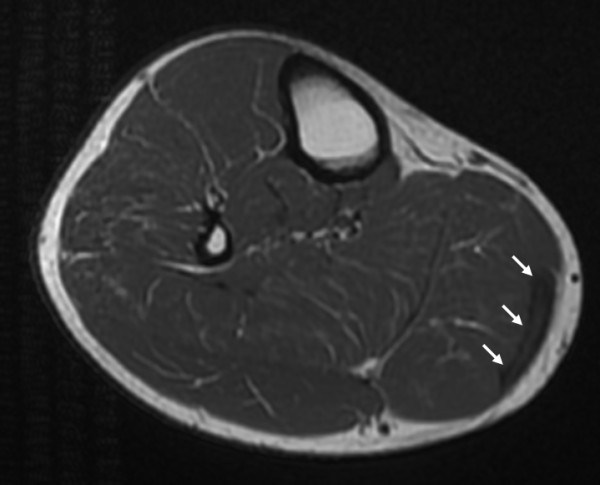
MRI of the right lower leg. Arrows pointing at a superficial hypointense lesion of proximal third of the medial gastrocnemic muscle.
